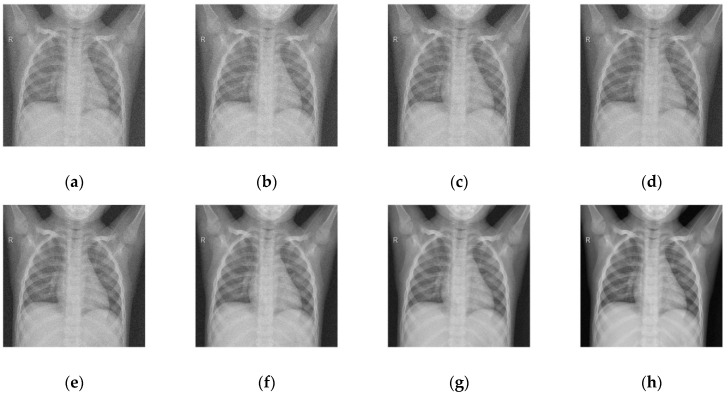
Denoised COVID-19 CT image 1 from the (a) [10], (b) [11], (c) [12], (d) [13], (e) [14], (f) [11], (g) [16], and (h) proposed frameworks, respectively.
